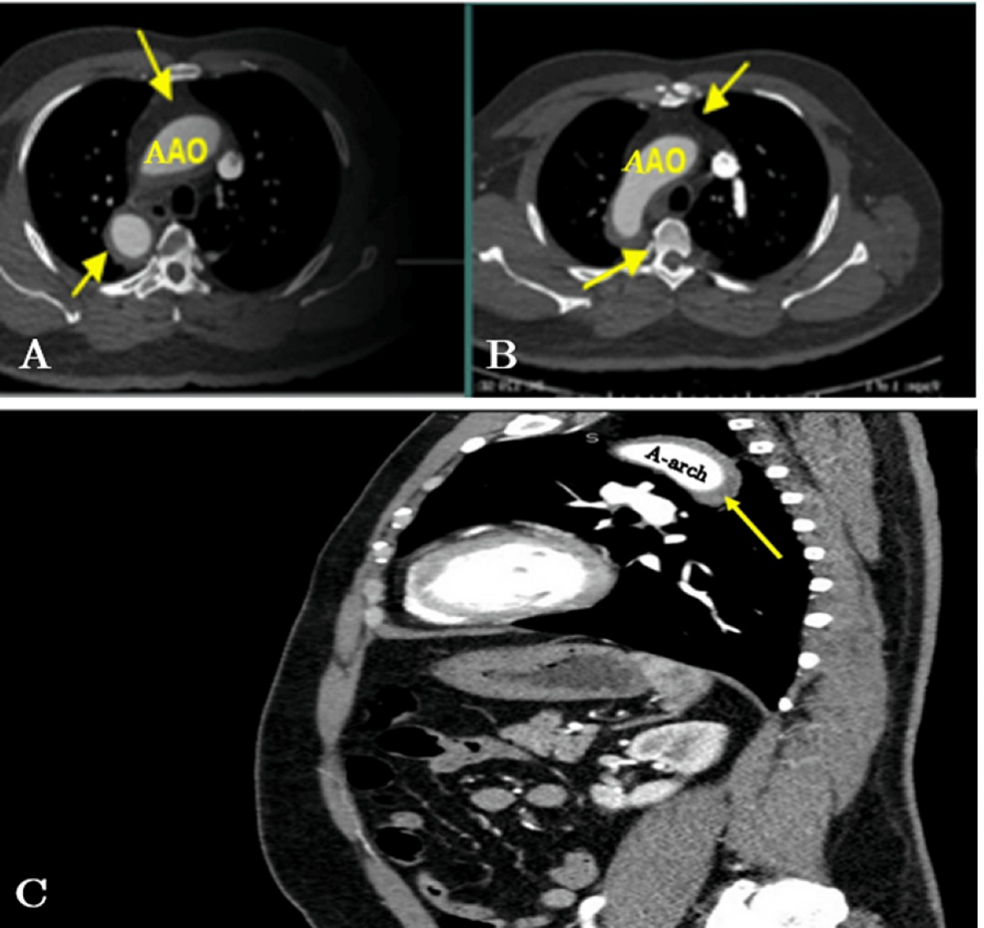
CT of the aorta with no radiological evidence of dissection or aneurysmal dilation (atherosclerotic changes). (A) Ascending aorta: intimal thickening of the aorta wall (yellow arrows). (B) Ascending aorta: intimal thickening of the aorta wall (yellow arrows). (C) Aortic arch: intimal thickening of the aorta wall (yellow arrow). Yellow arrows in A and B show the ascending aorta, while the arrow in C shows the aortic arch. CTA = computed tomography; AAO = ascending aorta; A-arch = aortic arch
